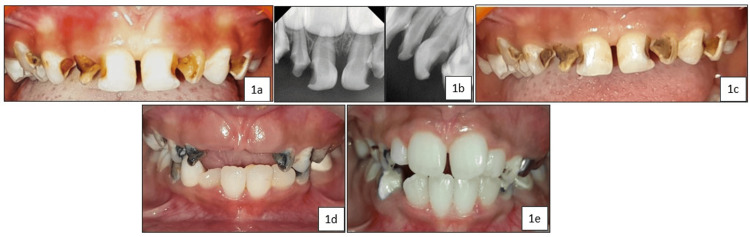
(a) Pre-operative image showing caries with an International Caries Detection and Assessment System II (ICDAS II) score of 5 in teeth 51, 52, 53, 54, 61, 62, 63, and 64; (b) Pre-operative radiograph showing deep dentinal caries in teeth 51, 52, 53, 54, 61, 62, 63, and 64; (c) Immediate post-operative image following silver diamine fluoride application; (d) 12-month follow-up showing exfoliated teeth 51 and 61; (e) 27-month follow-up showing erupting teeth 11, 12, 21, and 22.
